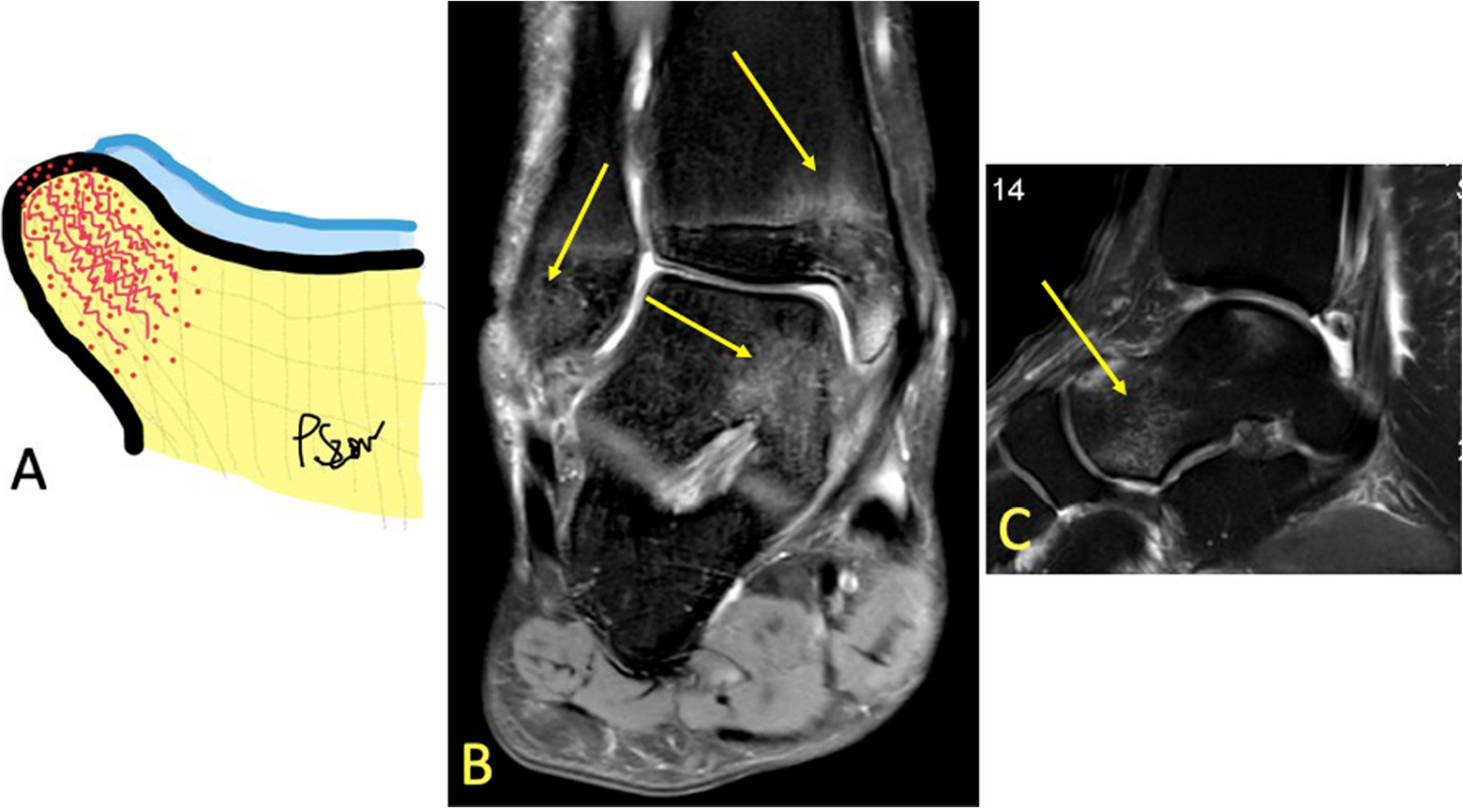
A 20-year-old soccer player presented after an ankle sprain during a soccer match with suspicion of a fracture in the lateral malleolus. MRI showed bone bruise in the tibia, fibula, and talus (arrows). It revealed no fracture of the lateral malleolus
